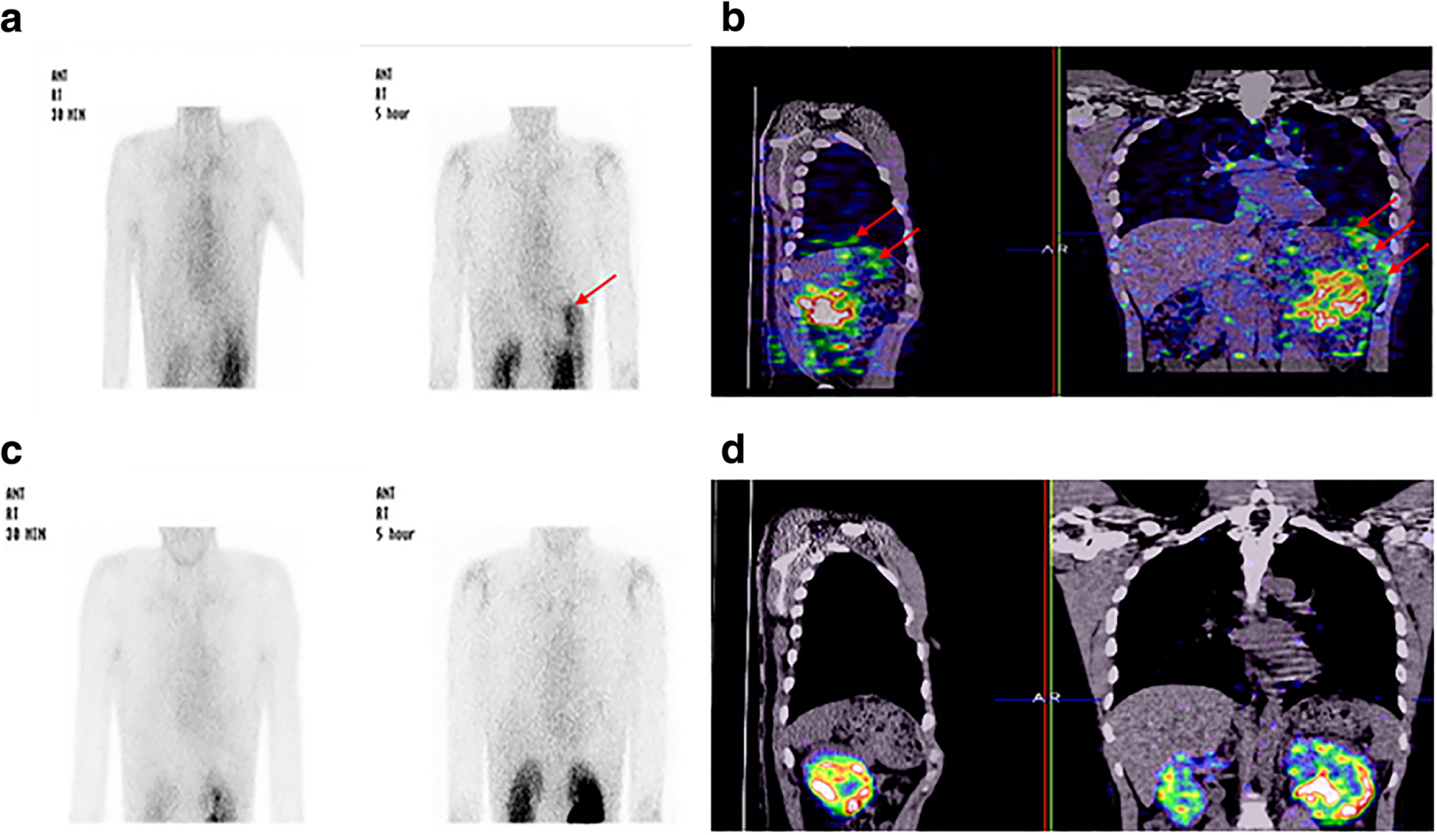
a Initial ^99m^Tc DTPA: Not 30 min, but 5 h posterior static image showing significant buildup of trace in the left sub-diaphragm and pleural space (arrow). b Initial SPECT-CT: Not 30 min, but 5 h scanning image demonstrating tracer uptake in the right sub-diaphragm and pleural space (arrow). c Follow up ^99m^Tc DTPA: 5 h posterior static image showing complete disappearance of buildup of trace in the right sub-diaphragm and pleural space. d Follow up SPECT-CT: 5 h scanning image demonstrating disappearance of tracer uptake in the right sub-diaphragm and pleural space
